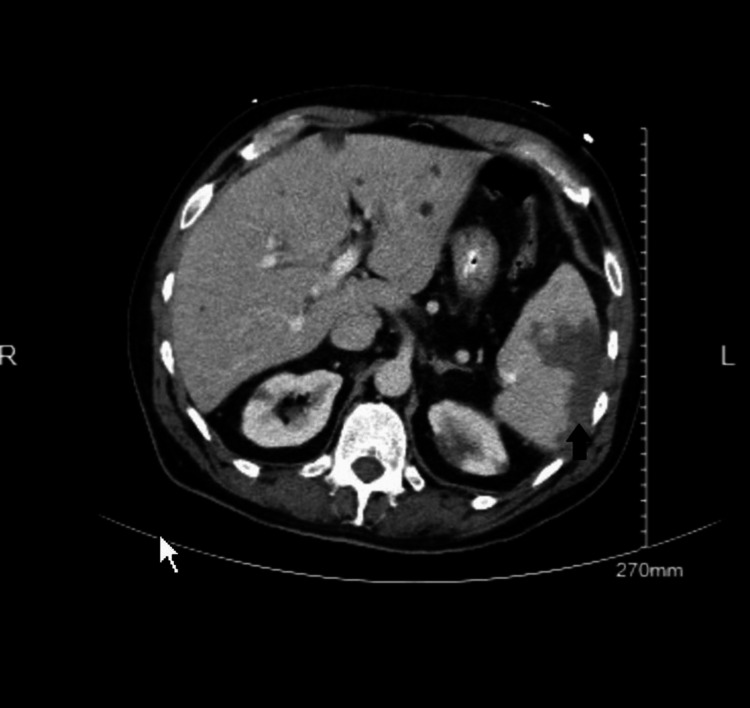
CT abdomen and pelvis demonstrate multiple wedge-shaped infarcts in the spleen.
